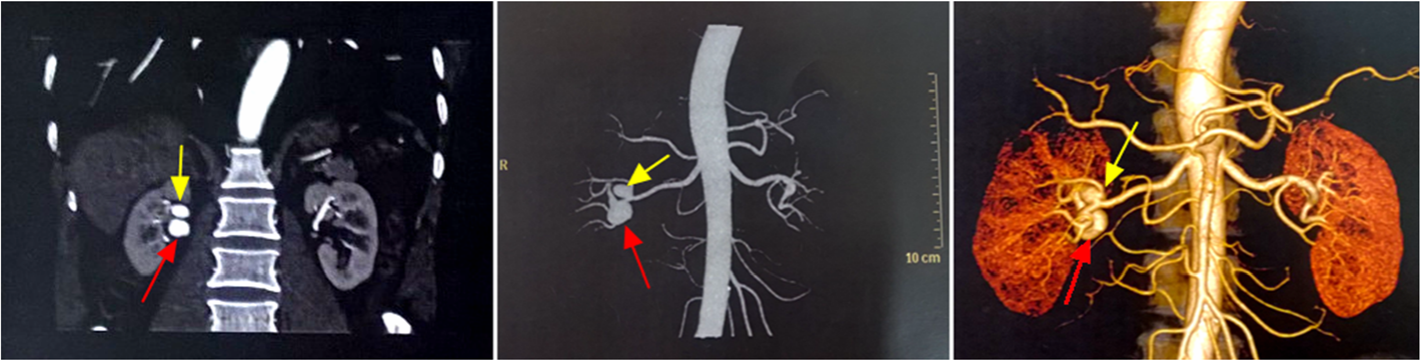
CT angiogram and reconstruction preoperative. Red asterisk indicates the RAA of 1.8 cm. Yellow asterisk represents the RAA of 1.2 cm
